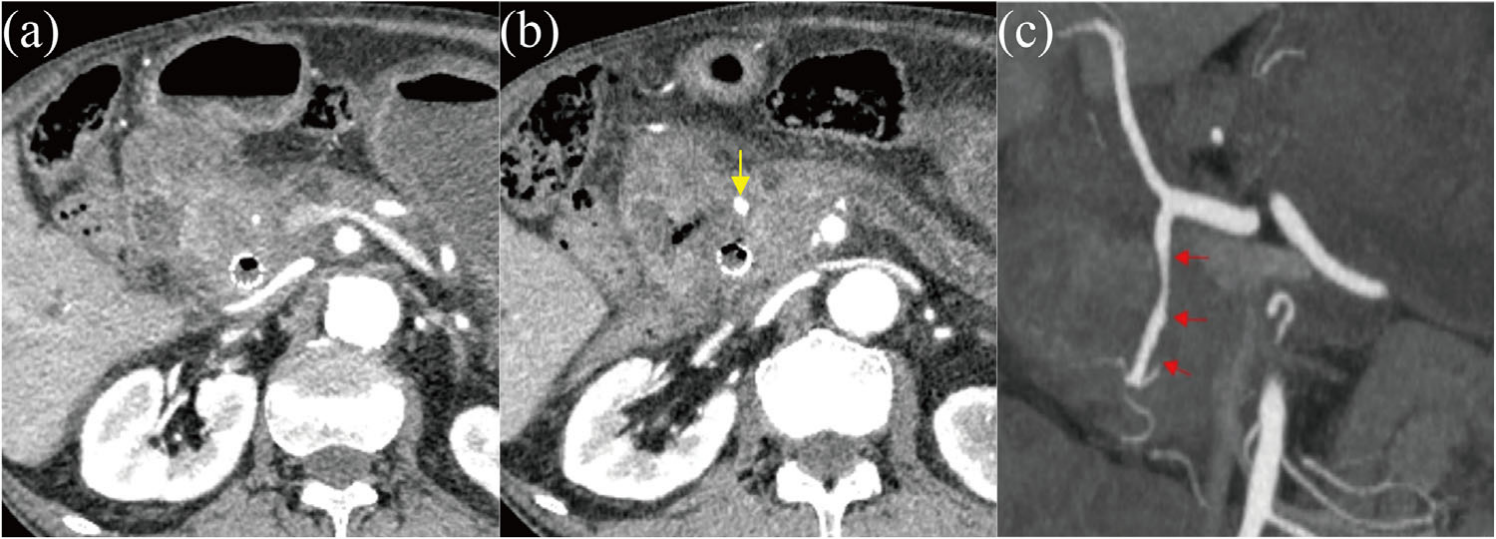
(a) Contrast‐enhanced computed tomography reveals narrowing in the gastroduodenal artery near the duckbill‐shaped anti‐reflux metal stent. (b) Contrast‐enhanced computed tomography reveals dilatation in the peripheral gastroduodenal artery compared to (a), considered to represent a pseudoaneurysm (yellow arrow). (c) Narrowing and irregular dilatation (red arrow) are found in the gastroduodenal artery and determined to represent pseudoaneurysm.
